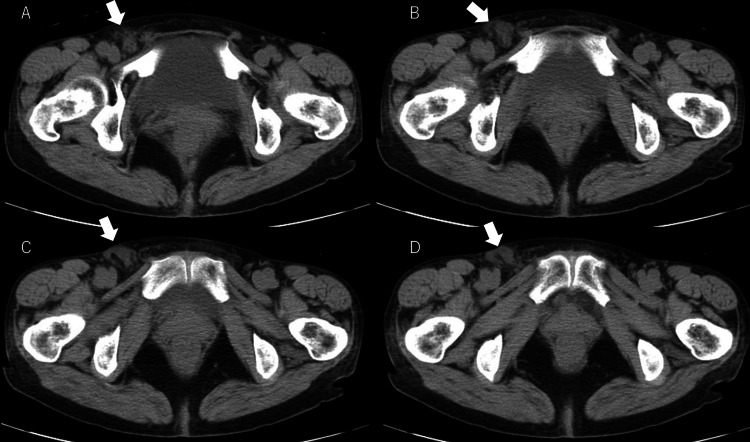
Plain abdominal CT scan after hydrocelectomy Plain abdominal CT scan revealing a right femoral hernia (arrows) CT, computed tomography
